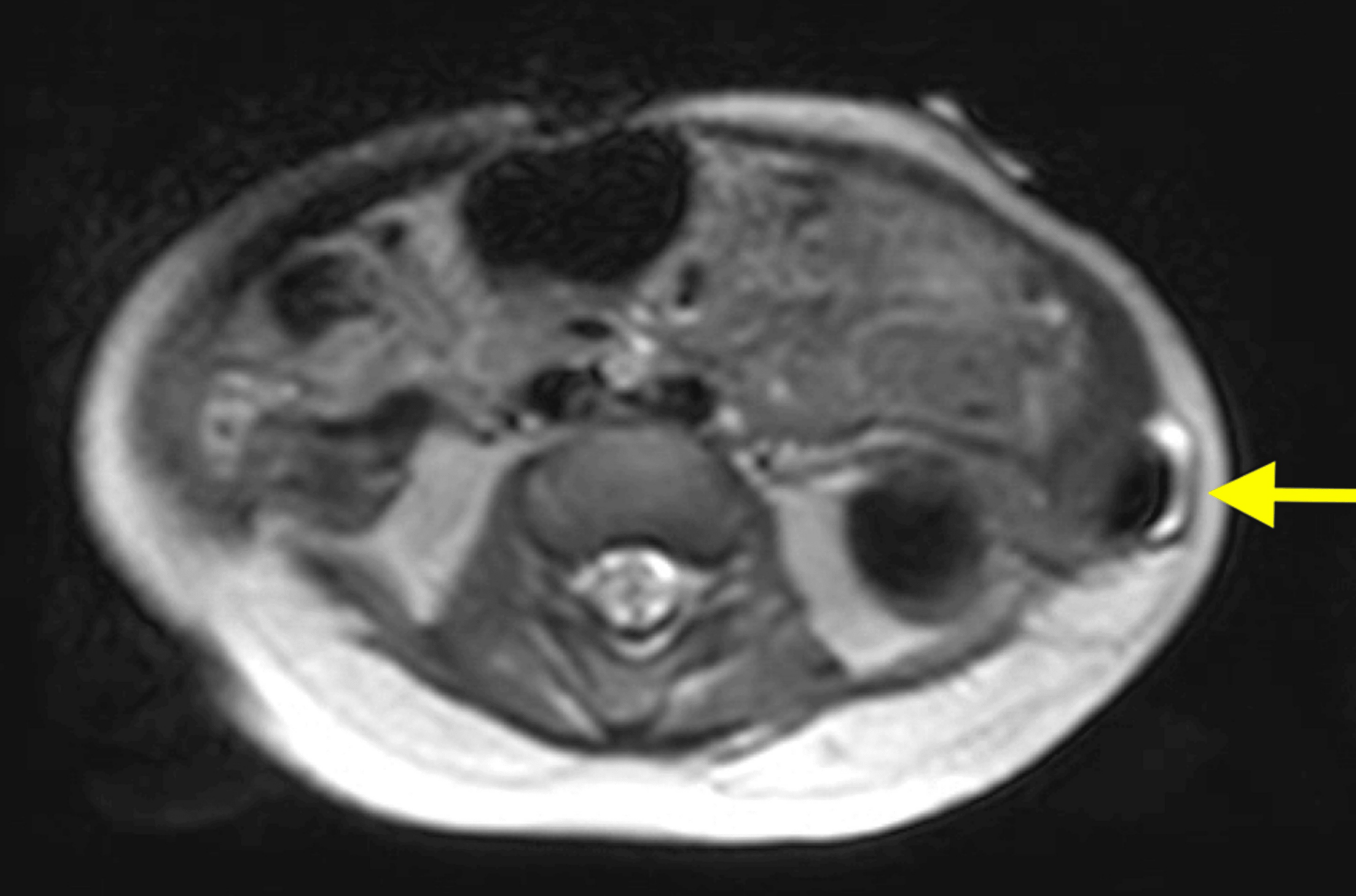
Axial view of T2-weighted MRI image demonstrating the left lumbar hernia containing bowel.
